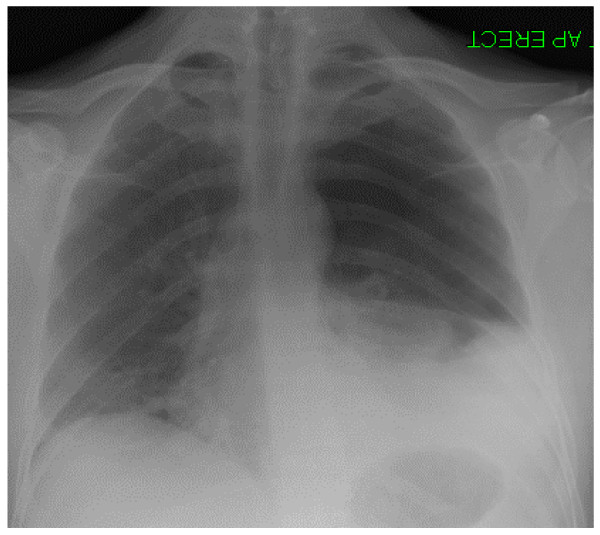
Erect chest radiograph showing pneumothorax occupying upper third of left lung field with some mediastinal shift and basal opacification.
